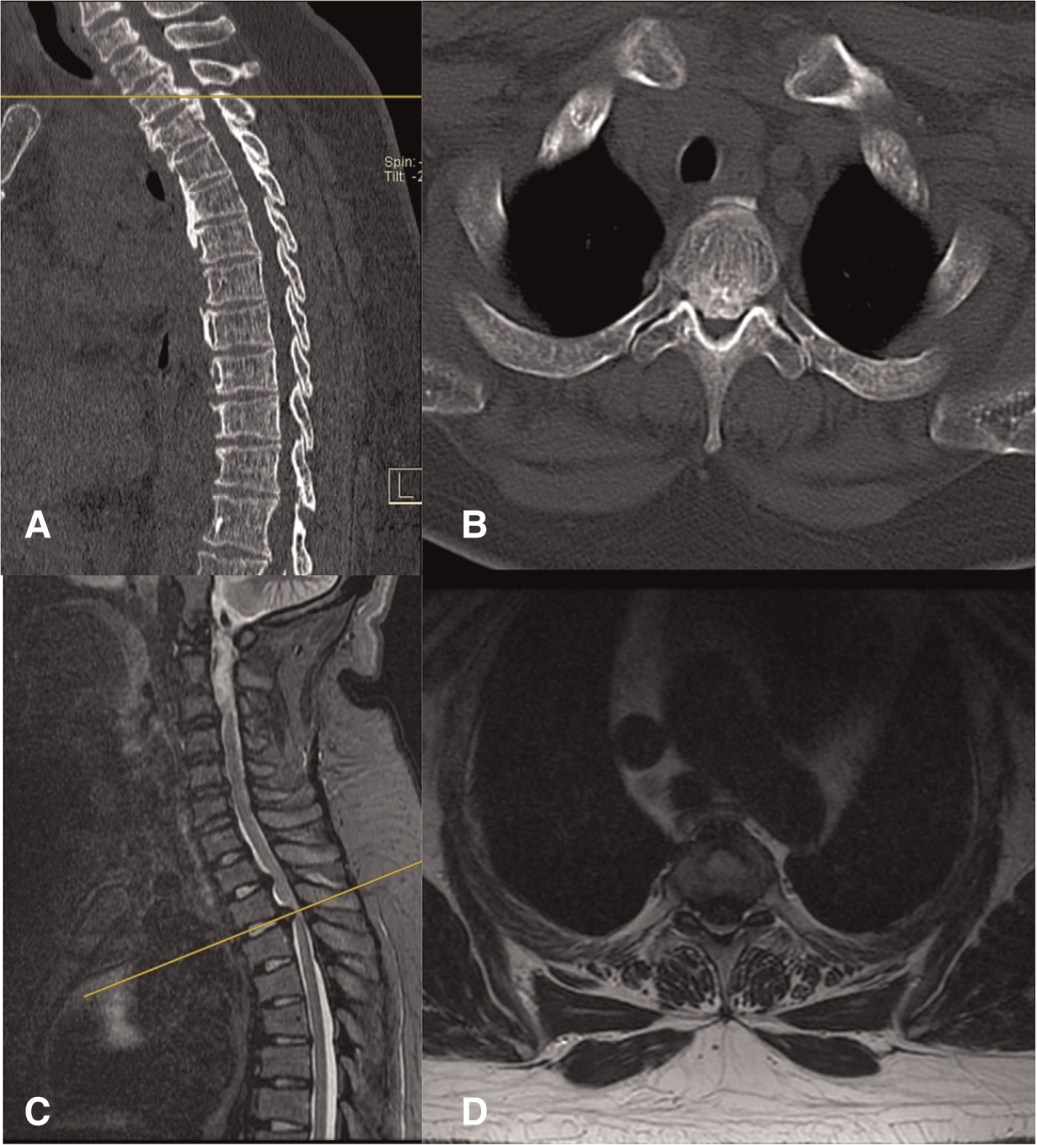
(A,B) The preoperative CT films conformed TOPLL at T1–T2 and T2–T3. (C,D) The preoperative MRI suggest the spinal cord at T2–T3 was compressed by TOPLL and became thinning.
